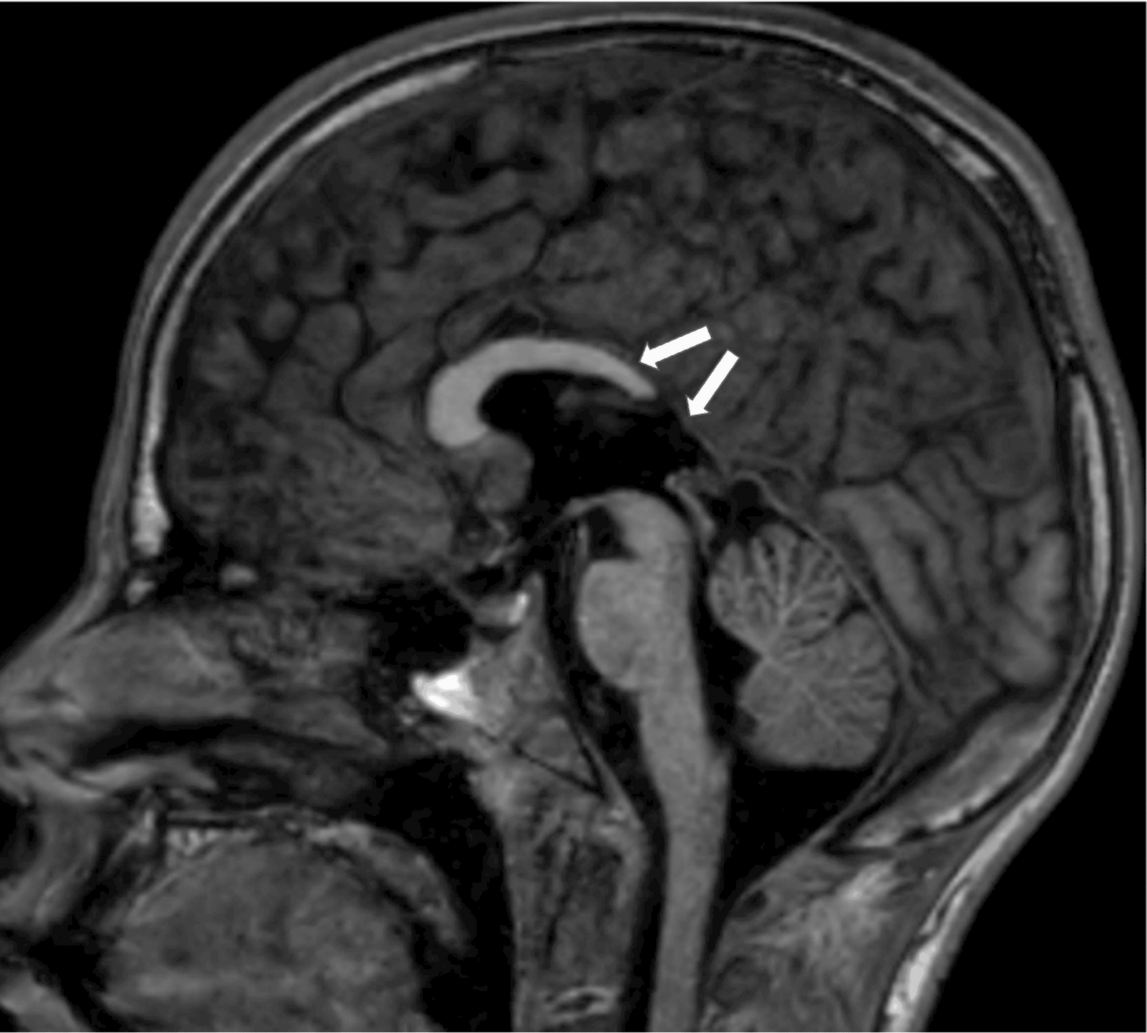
Sagittal T1-weighted MRI of the brain without contrast shows dysgenesis of the corpus callosum and agenesis of the dorsal body and splenium (white arrows) T1 - longitudinal relaxation time
